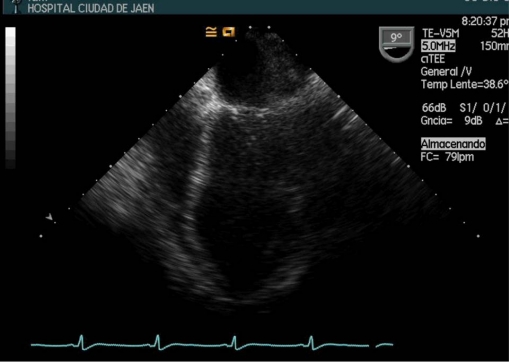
Transesophageal image projection which displays the left ventricle of a 18-year-old female patient with urinary sepsis by *E. colli*. The arrow points to "*ballooning*" septoapical segment.
